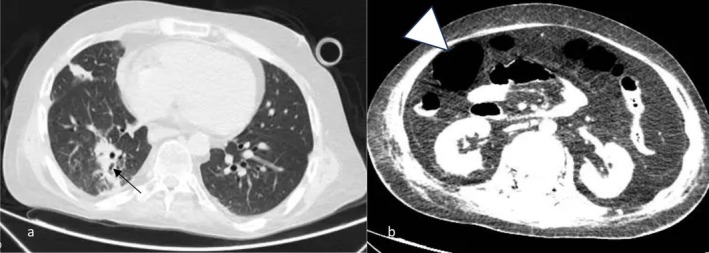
(a) Spiral chest computed tomography (CT) scan indicating bronchiolitis obliterans syndrome and right lower lobe consolidation (arrow), (b) Spiral abdomen‐pelvic CT scan showing dilated bowel loop (arrowhead) in favor of ileus.
